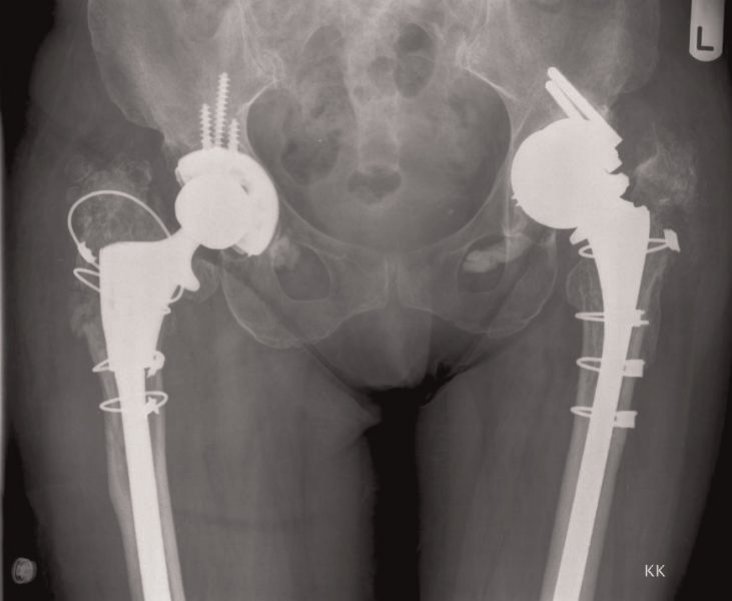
Radiograph at 12^th^ month postoperative period showing a posterior inferior migration of acetabular cup in AP view.
